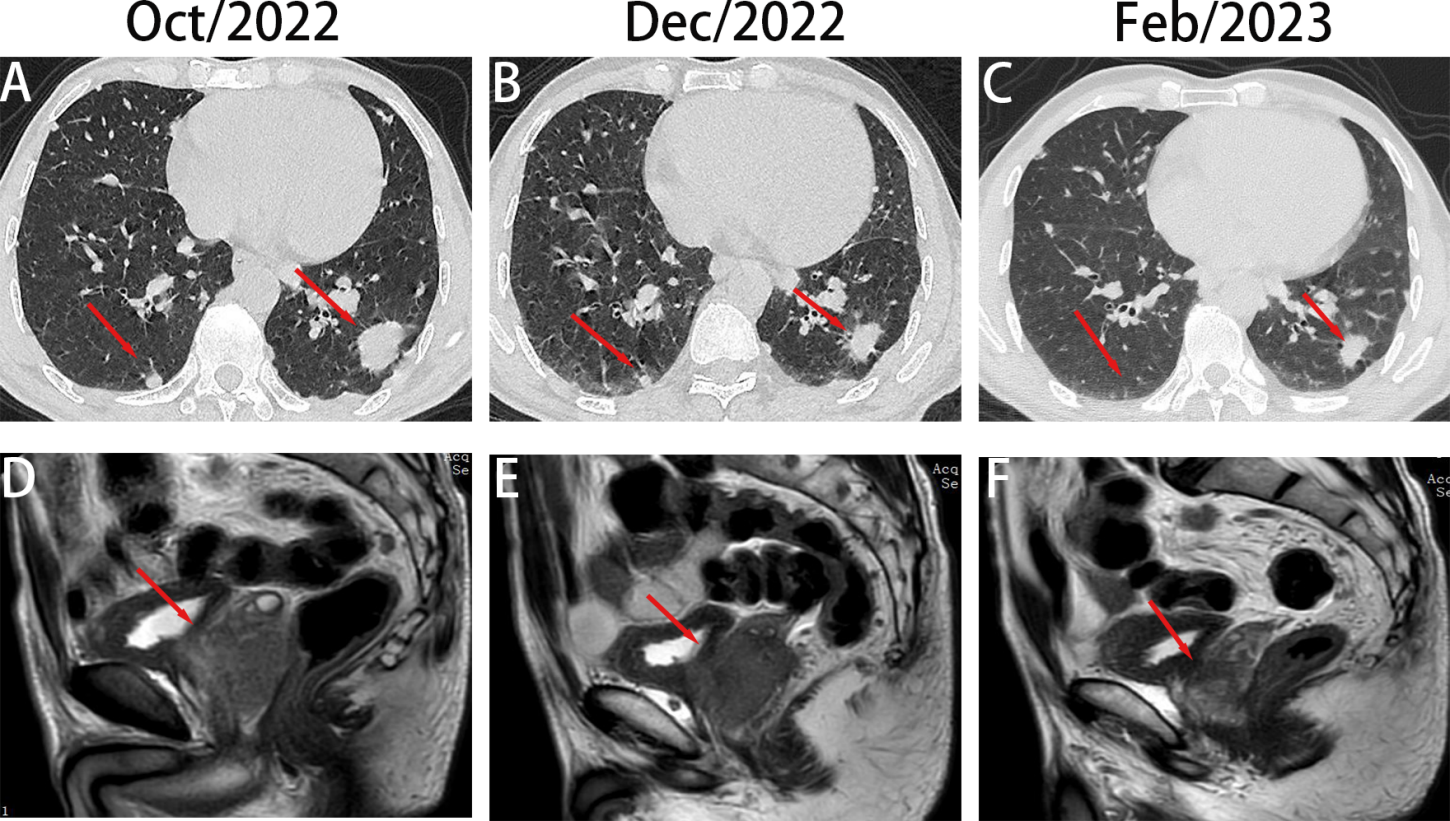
Chest computed tomography (CT) and prostate magnetic resonance imaging (MRI) scan of the patient throughout the course of diagnosis and treatment. (A–C), Imaging changes of chest CT (mediastinal window and pulmonary window) and prostate MR at different time points (D–F).
